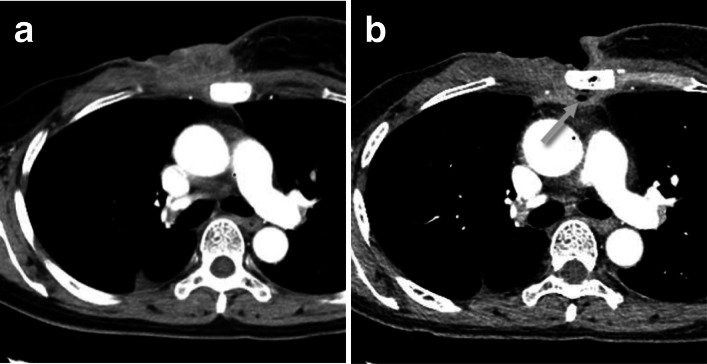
CT findings of the tumor in the chest wall. a A CT image obtained before the administration of everolimus plus exemestane. b A CT image obtained when the patient experienced fever during treatment with everolimus and exemestane. An arrow indicates an air bubble
